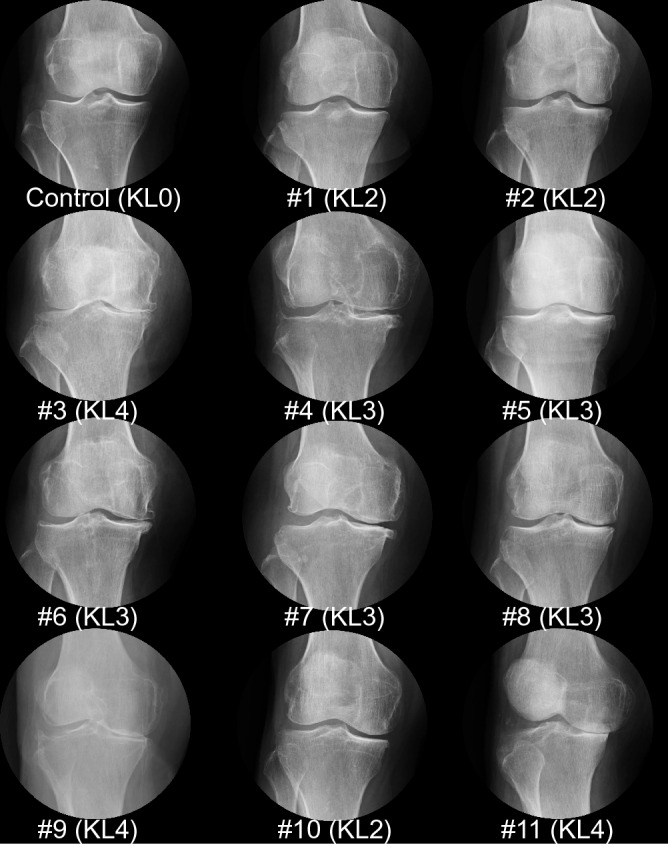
Anteroposterior radiographic images of the knee in the standing position. The images are ordered from highest to lowest in terms of their cartilage area ratios at the medial tibia. The Kellgren–Lawrence grade is also shown.
